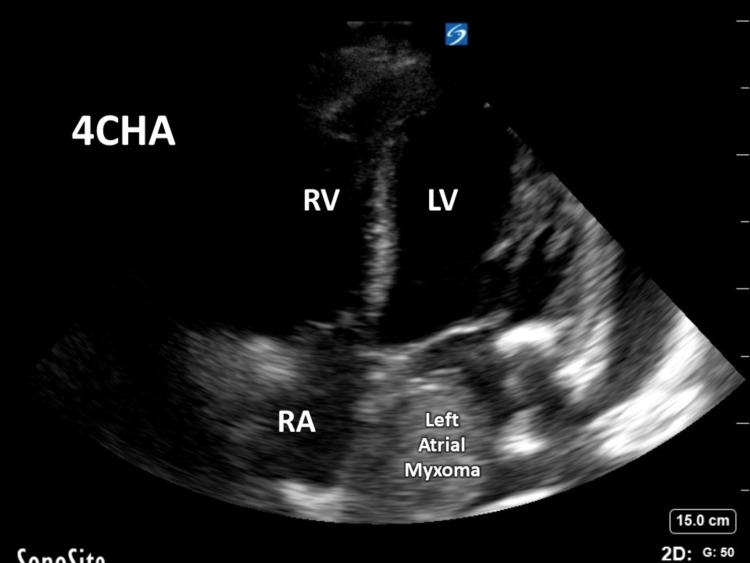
Transthoracic echocardiogram (TTE) left atrial myxoma 4-chamber view 4CHA - 4 chamber, LV - left ventricular, RV - right ventricular, RA - right atrium
